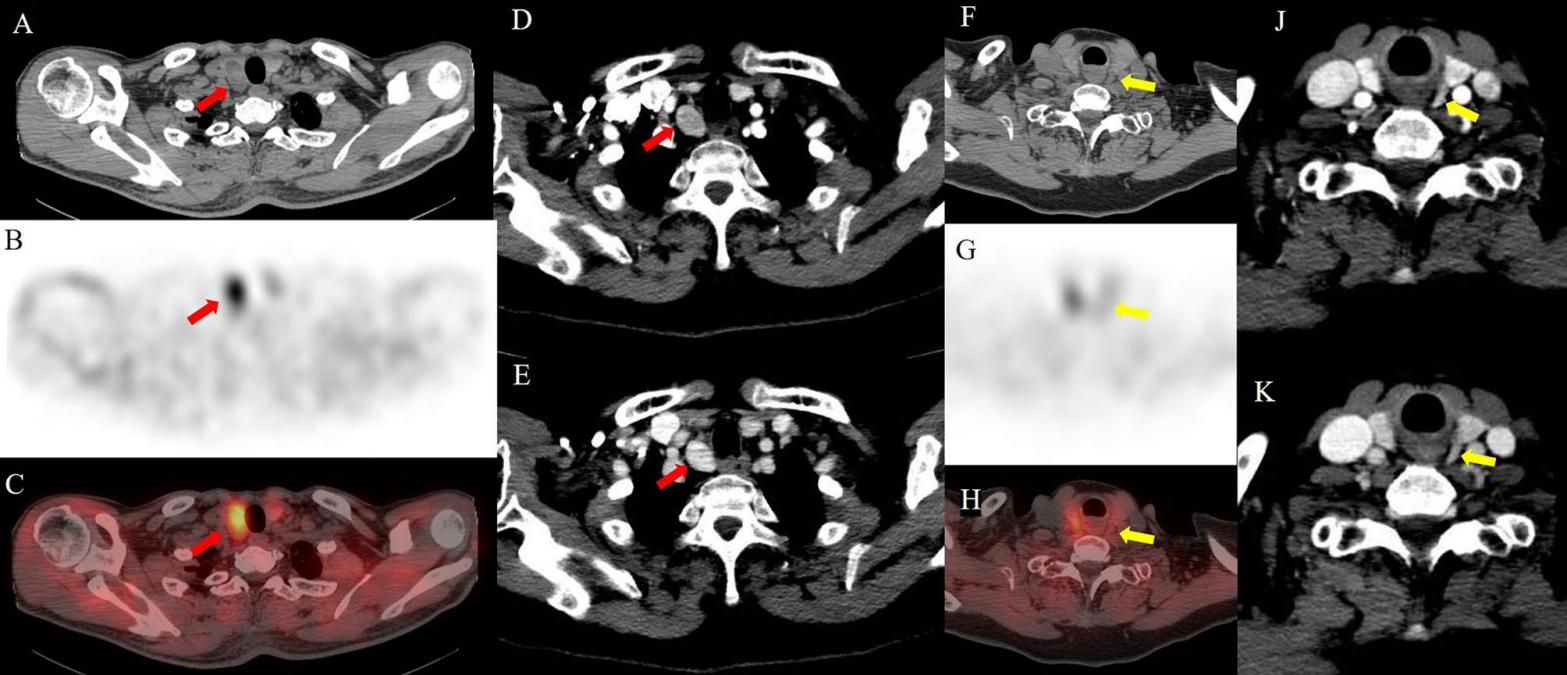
Image of an 83-year-old woman with primary hyperparathyroidism. Sestamibi SPECT/CT images (A, axial noncontrast-enhanced; B, axial SPECT; C, fusion SPECT/CT) reveal focal radioactivity uptake localized to a single abnormal right inferior parathyroid gland (red arrows). 4D-CT (D, arterial phase; E, delayed phase) shows a vividly enhancing lesion at the arterial phase and persistence of contrast material with increasing attenuation compared with the atrial phase at the delayed phase (red arrows). An additional left superior parathyroid gland (yellow arrows) was observed on noncontrast-enhanced CT (F), which revealed no focal uptake on sestamibi SPECT/CT (G, axial SPECT; H, fusion SPECT/CT), but mild moderate enhancement was noted on 4D-CT (J, arterial phase; K, delayed phase). The patient underwent bilateral four-gland exploration for left superior parathyroid hyperplasia and right inferior parathyroid adenoma. Normal PTH and calcium levels were observed after 26 months of follow-up
